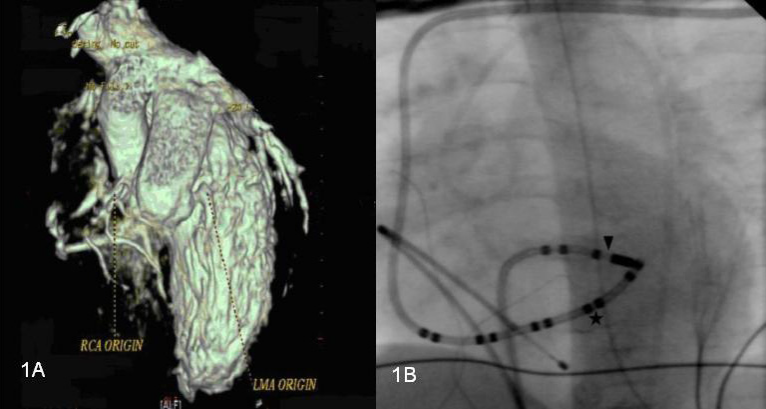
(A) Multi-slice computed tomographic image of the anomalous origin of the left main coronary artery from the pulmonary artery, and (B) location of the successful ablation site at the left posterolateral area. Shown are the coronary sinus catheter (*) and the ablation catheter (inverted filled triangle).
